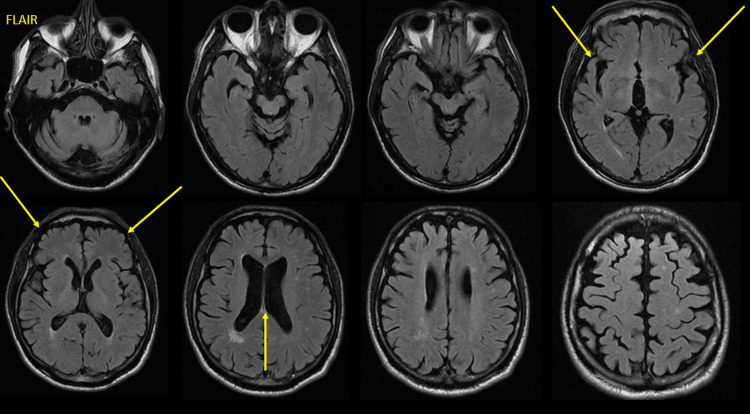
Brain MRI in FLAIR sequence, where cortical and subcortical atrophy is shown, predominantly in the frontal and temporal lobes, along with compensatory ventriculomegaly. FLAIR: fluid-attenuated inversion recovery
